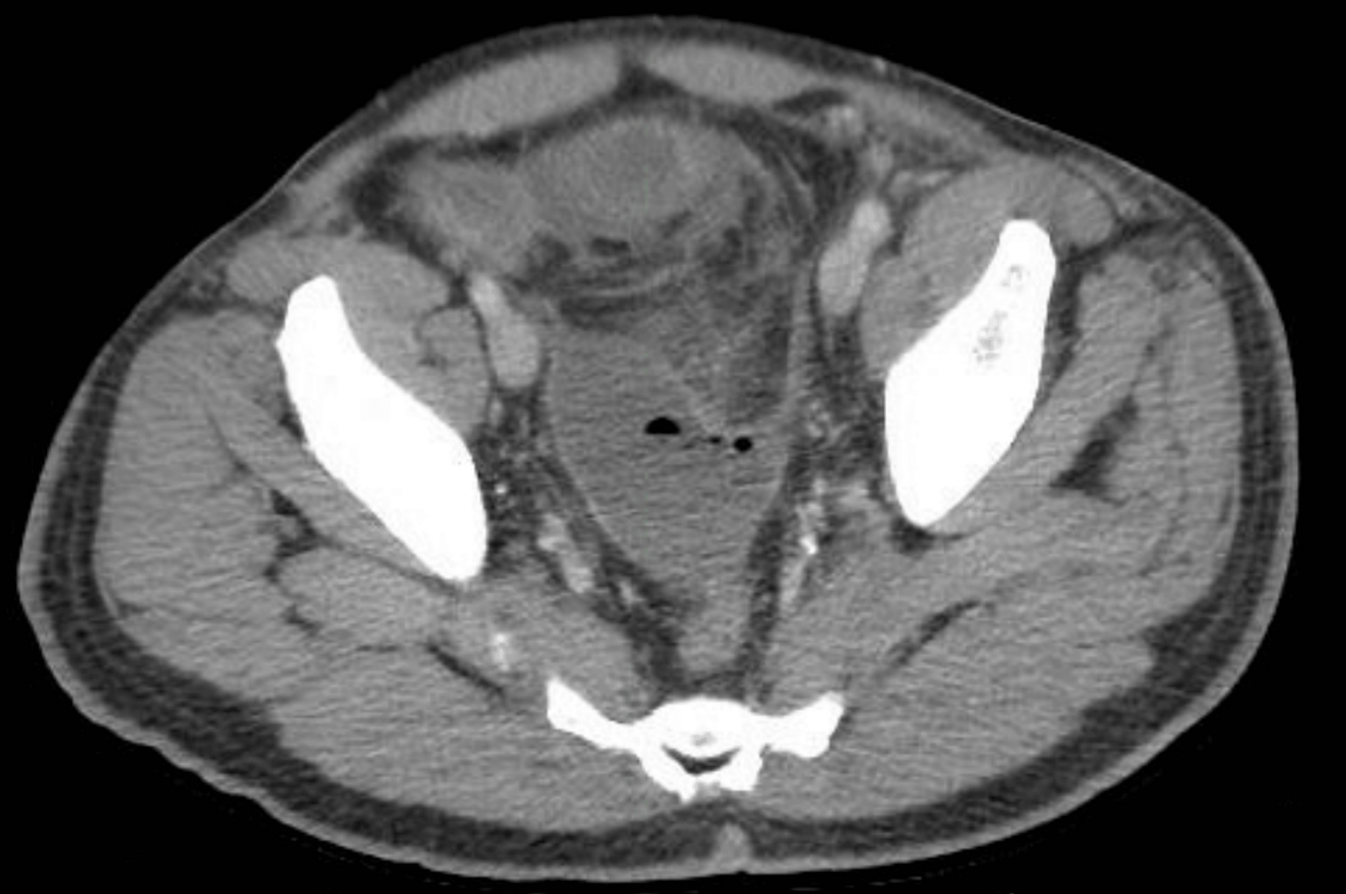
Axial contrast-enhanced CT image demonstrating a pelvic fluid collection with internal gas locules, measuring approximately 6.6 × 3.8 × 7.4 cm, findings concerning for a pelvic abscess in the setting of small bowel obstruction and inflammatory ileitis.
